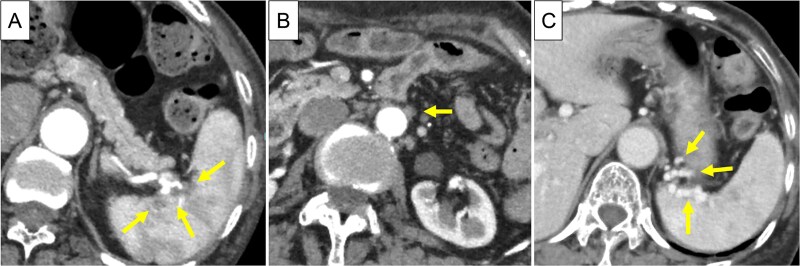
CE-CT after chemotherapy. (A) The tumor in pancreatic tail had shrunk to 3 cm in size (arrows). (B) The para-aortic lymph nodes were shrunk (arrow). (C) Collateral veins were amplified within the posterior wall of the upper gastric body (arrows).
